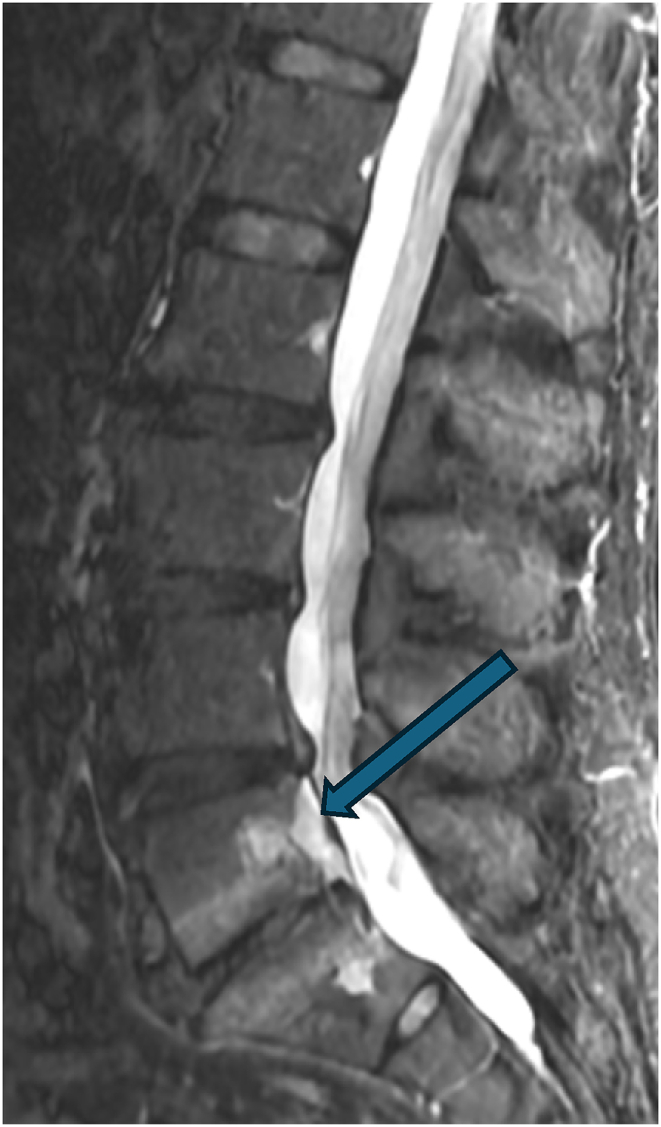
Sagittal STIR MRI 8 months post-procedure. Arrow demonstrates hematoma posterior to the L5 vertebral body.
